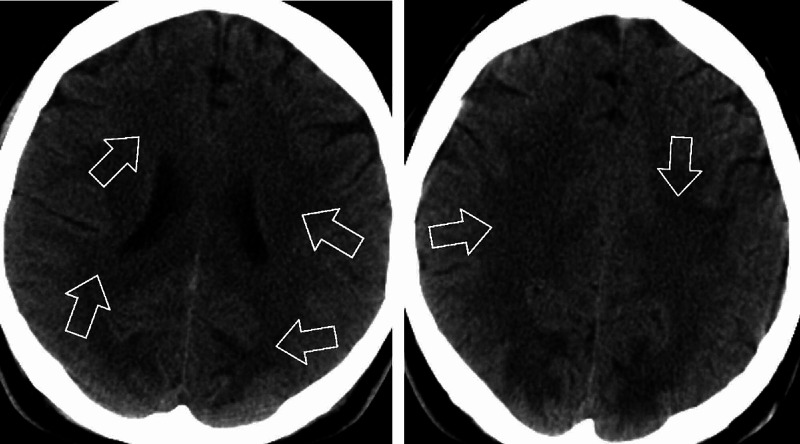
CT head without contrast
